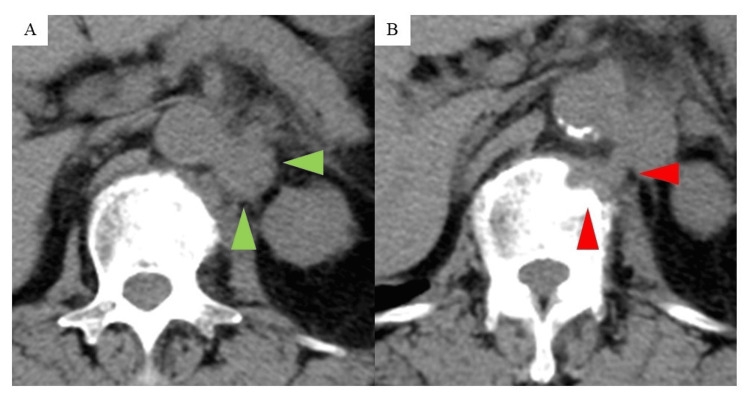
CT scan four months after discontinuing pembrolizumab treatment A: Green arrowheads show lymph nodes. B: Red arrowheads show bone metastasis. CT: computed tomography
